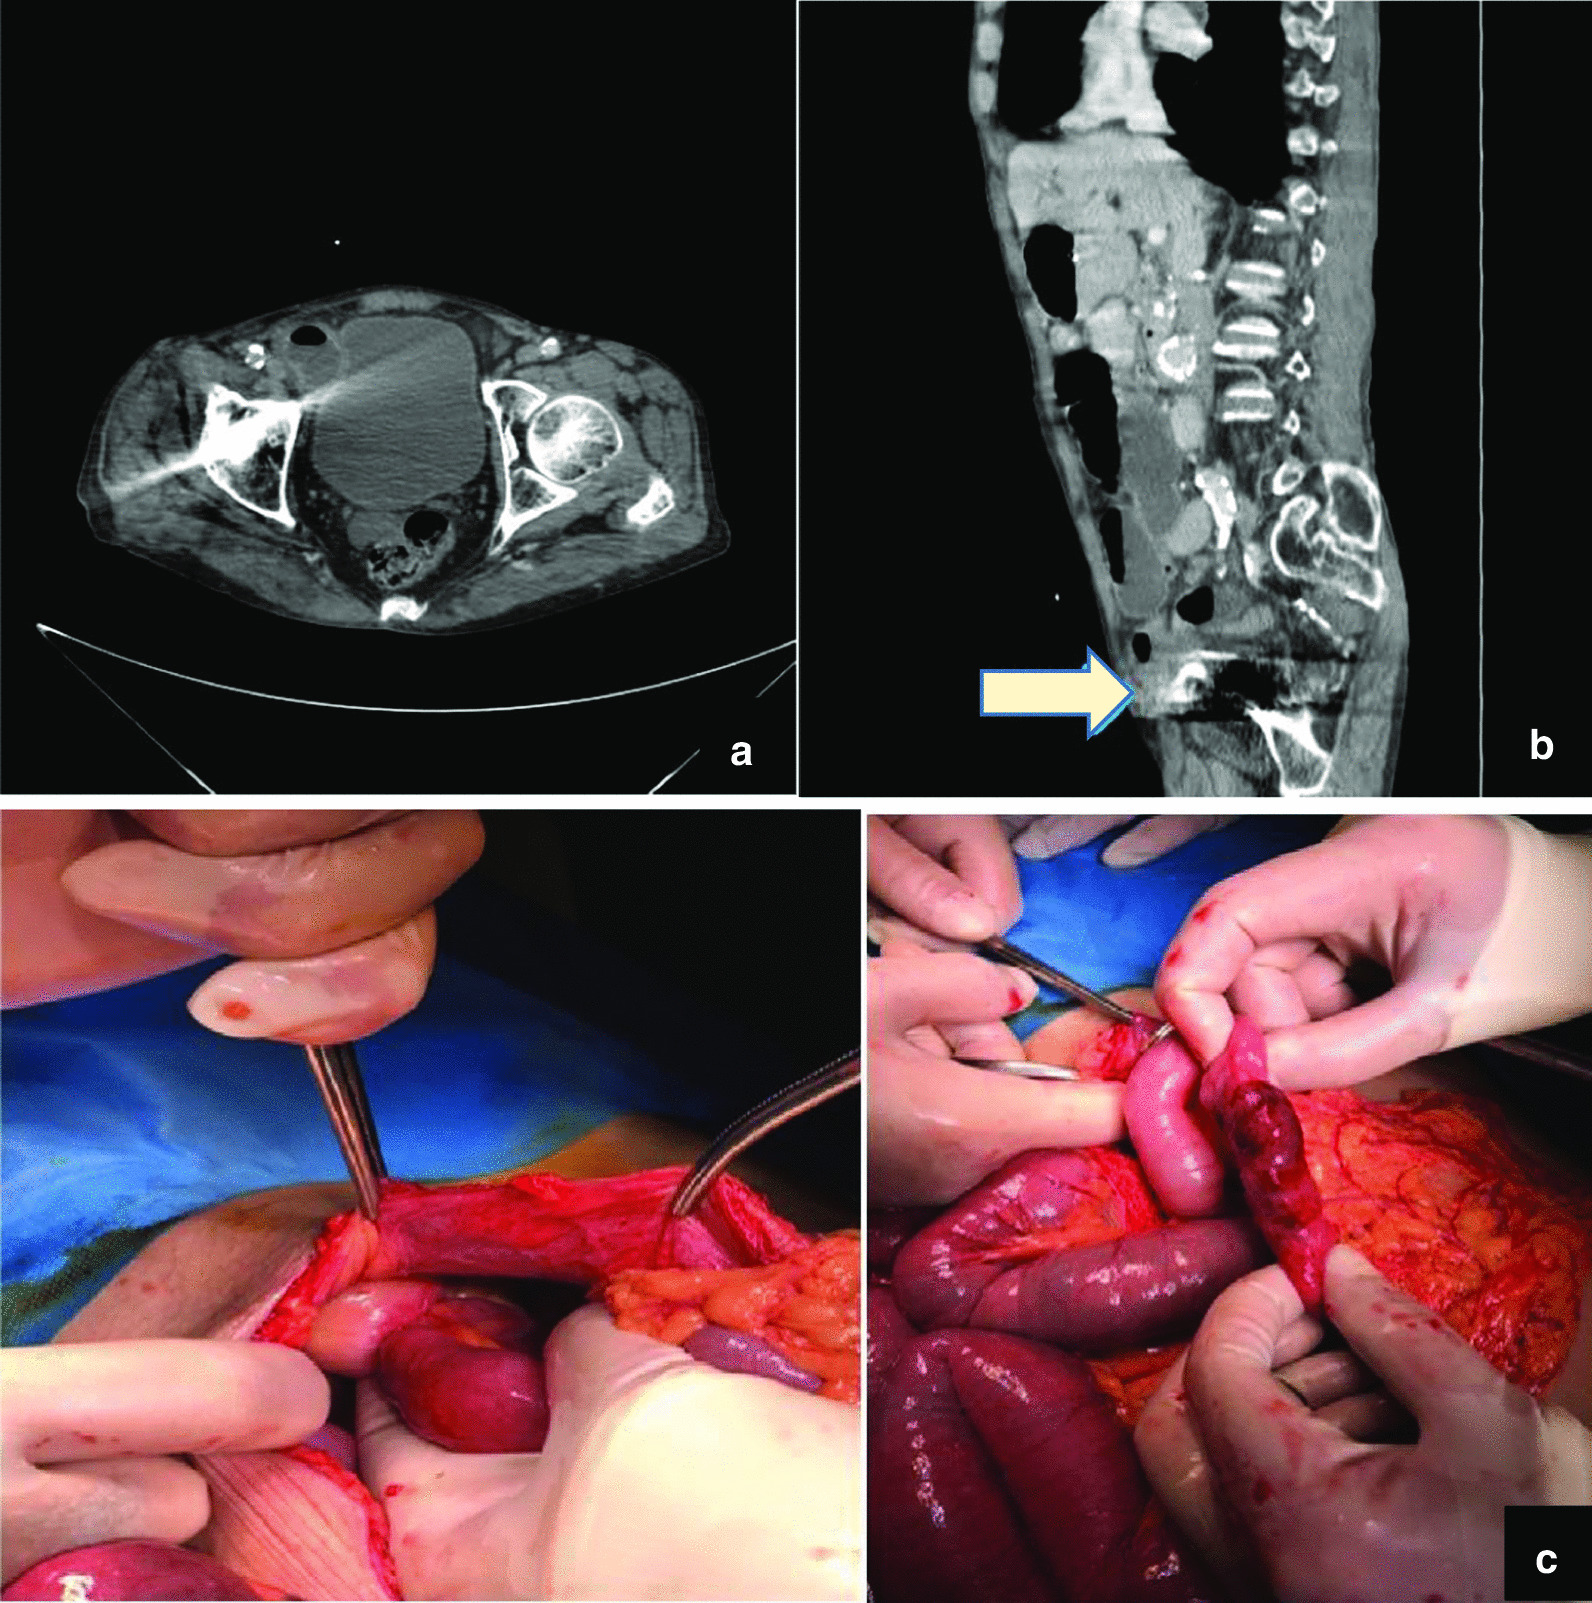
a Computed tomography scan of the abdomen and pelvis, b obstruction of small intestine and hernia sac and its contents inside the abdominal wall, c small intestinal loop with slight discoloration and adherence to the wall of the hernia sac inside the inner ring of the inguinal canal. The arrow in computed tomography indicates the hernia sac (under the fascia)
